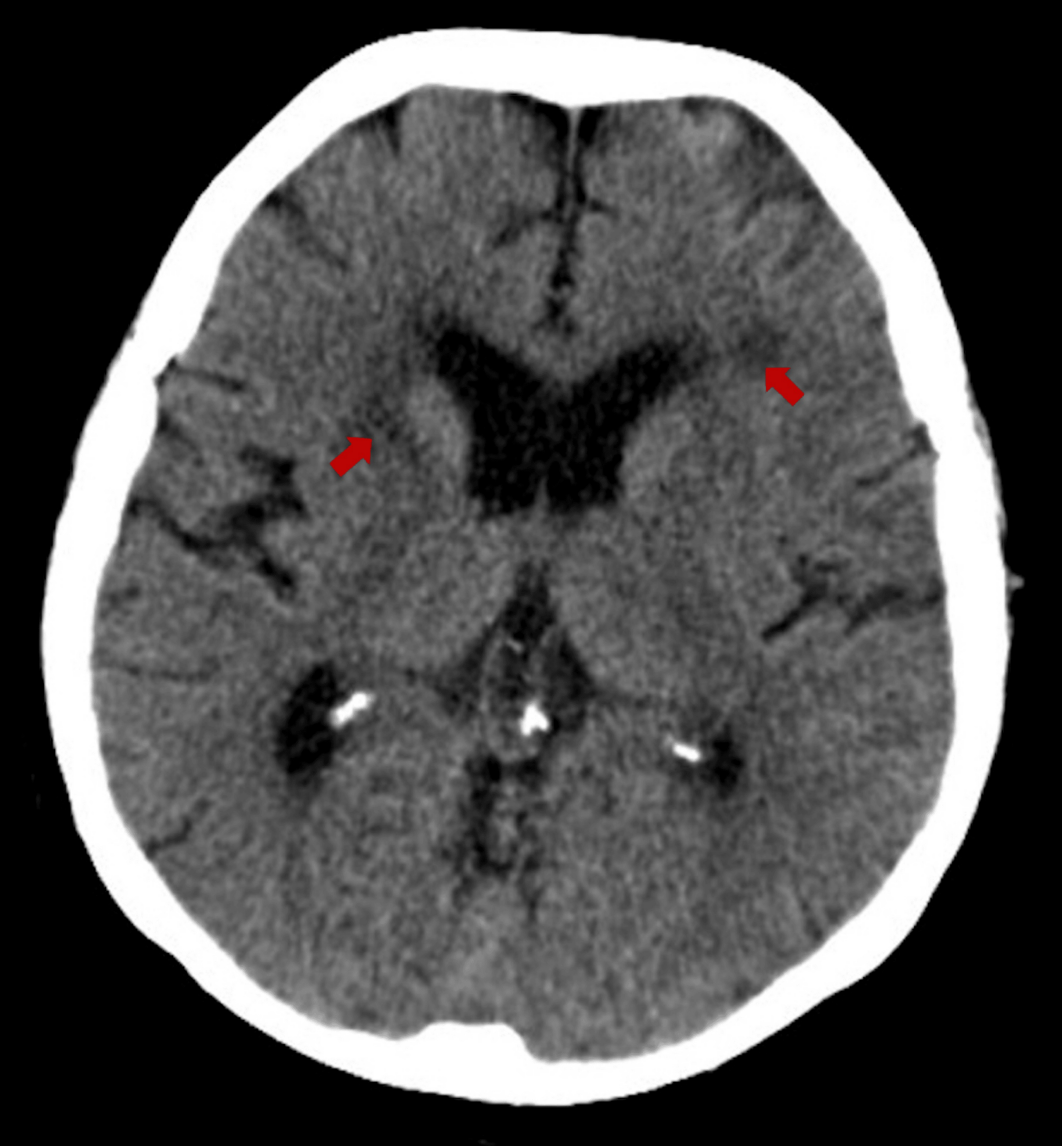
CT head without contrast, performed in the emergency department. Findings shown: Periventricular microvascular ischaemic changes with no acute haemorrhage or large artery territory infarction.
